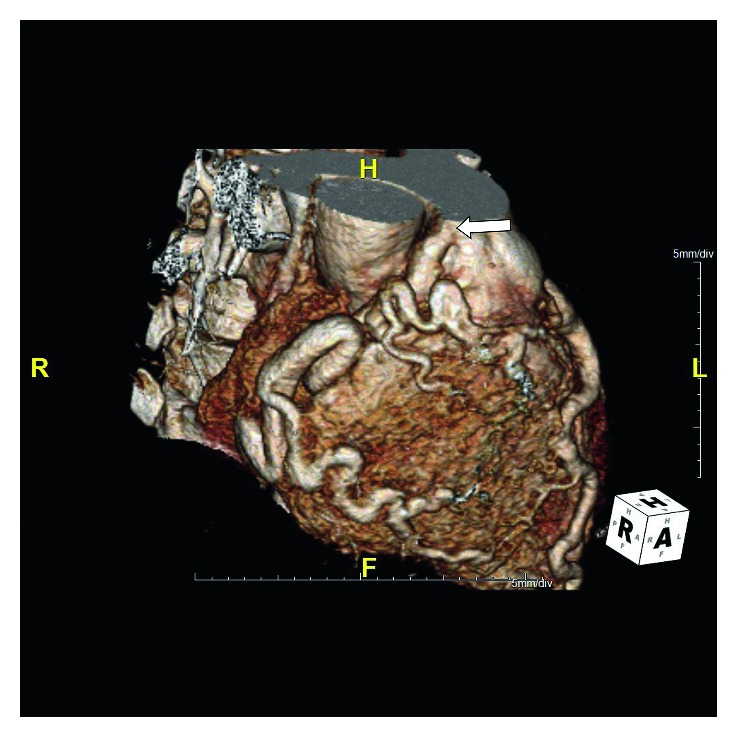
Coronary computed tomography angiogram (volume rendered image) demonstrates another view of the anomalous origin of the right coronary artery (RCA) from the pulmonary trunk (white arrow).
